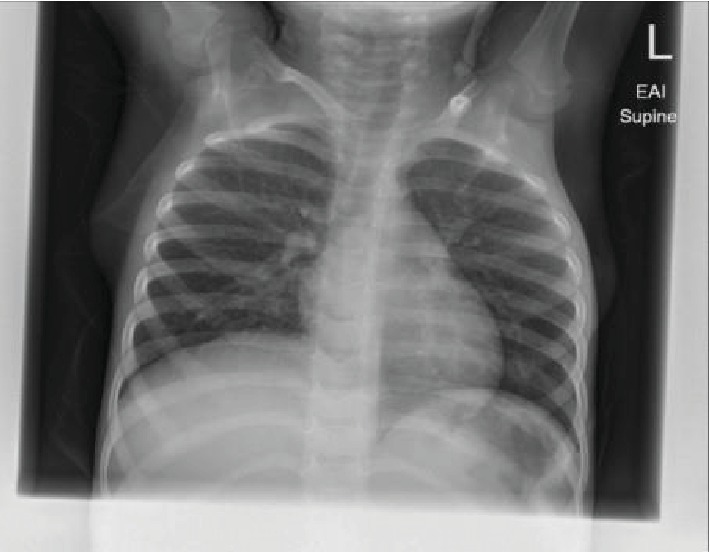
Chest X-ray showing an abdominal mass.
